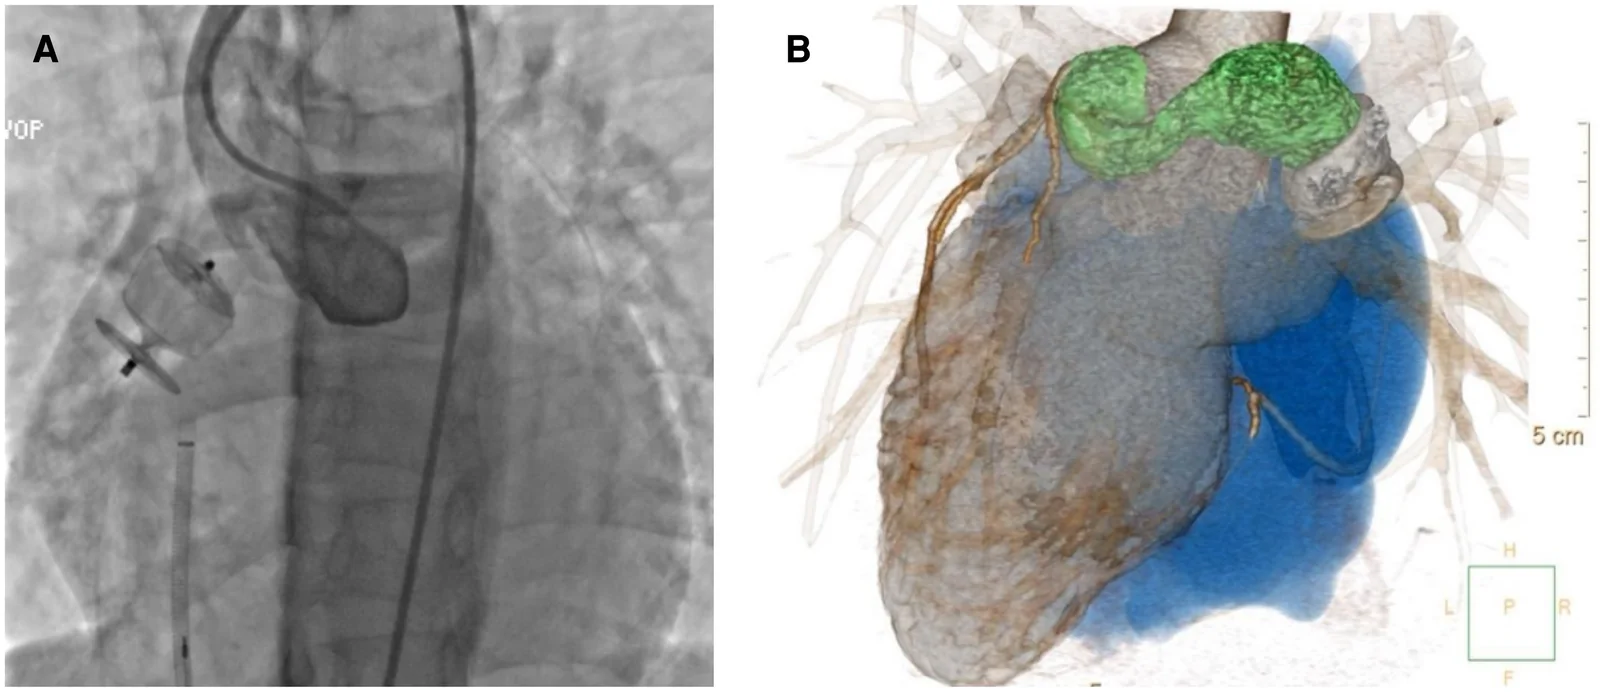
Percutaneous closure of the left coronary trunk (LCT) to right atrium (RA) fistula using a 20 mm amplatzer vascular plug II (AVP II), deployed on the RA side of the fistula. (*A*) Anterior view on coronary angiography demonstrating device placement. (*B*) Posterior view from 3D cardiac CT scan confirming the position of the vascular plug within the fistulous tract.
